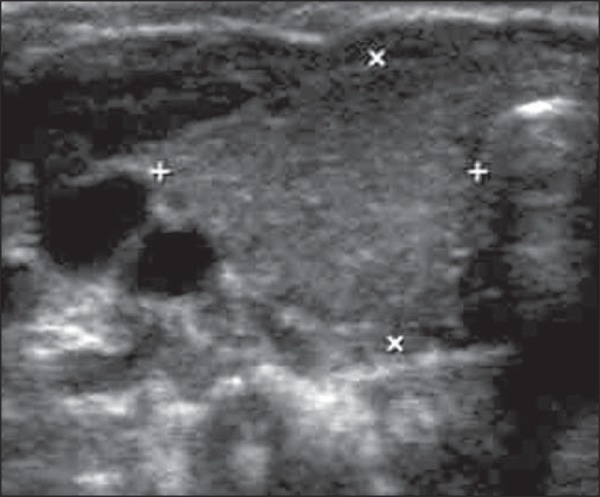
Ultrasonography of the cervical region in an asymptomatic child. Observe the right
